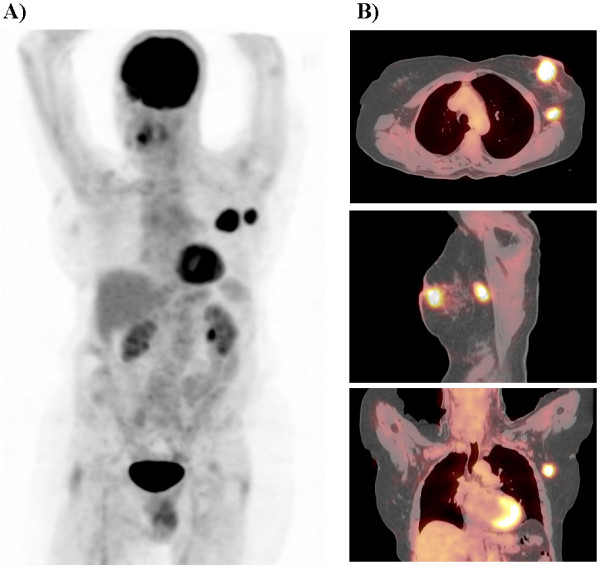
Preoperative PET maximum intensity projection in the lateral projection view (A) and cross sectional fused PET/CT images (B). The preoperative PET/CT scan revealed two hypermetabolic foci, representing the left breast primary tumor and the solitary left axillary metastasis.
